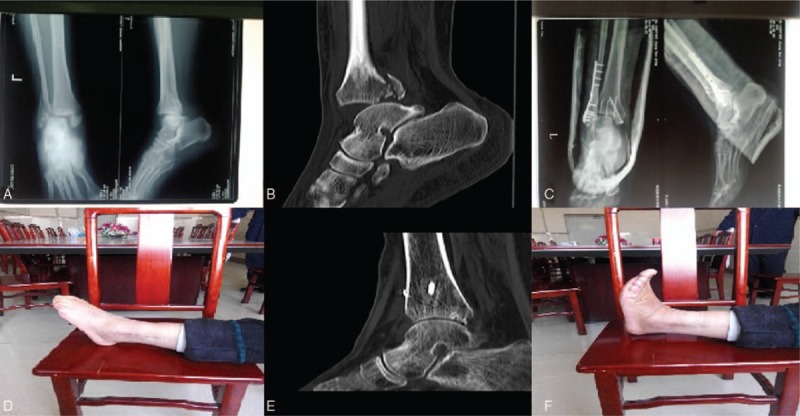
X-ray photograph and CT examination of one patient before and after the ankle joint dislocation. (A) X-ray photograph before the ankle joint dislocation; (B) CT examination before the ankle joint dislocation; (C) X-ray photograph after the ankle joint dislocation; (D) ankle flexion activity one year after the ankle joint dislocation; (E) CT examination 7 months after the ankle joint dislocation; (F) ankle extensor activity one year after the ankle joint dislocation. ∗The patient was female and 58 years old. Sprain led to swelling and pain of right ankle and she was admitted to hospital 2 day after limiting of activities. CT = computed tomography.
